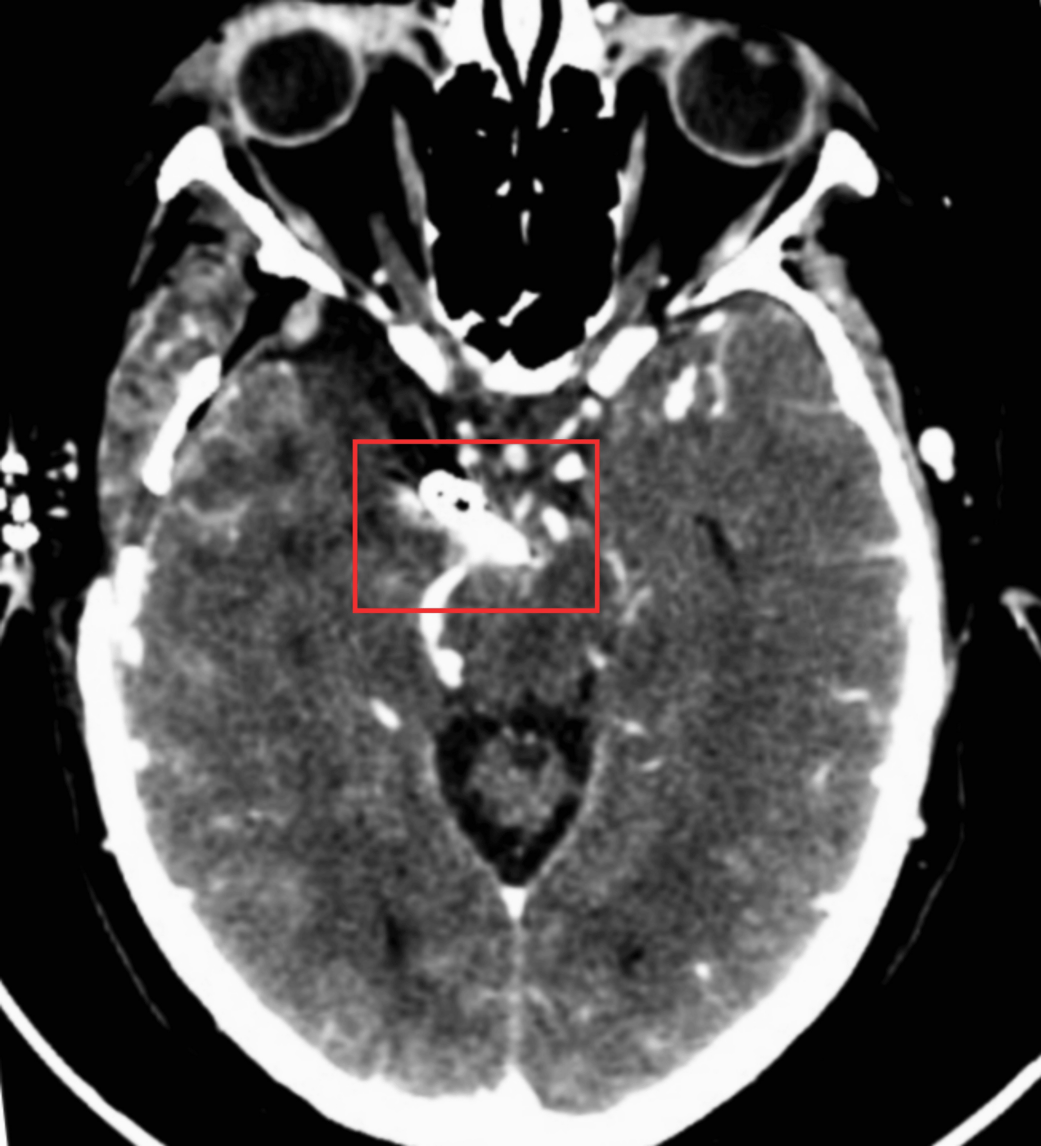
Postoperative control cranial CT scan showing an aneurysm clipping at the basilar artery bifurcation (red square).
